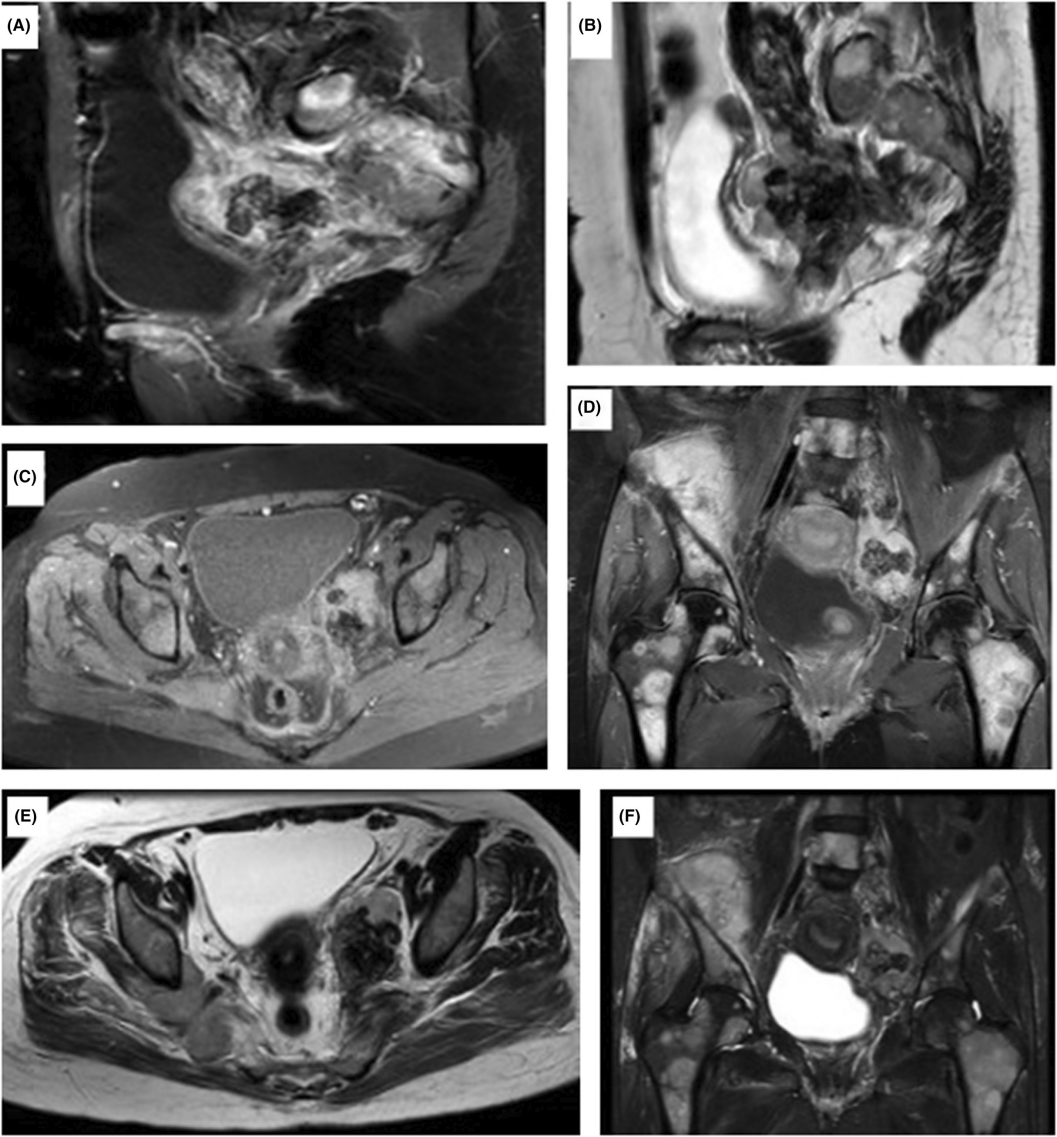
Coronal (A) and sagittal (C) contrast‐enhanced images revealed peripheral moderate enhancement of the non‐calcified components of the mentioned retroperitoneal mass and its extension to the left iliac vein complex as well as multiple enhancing bony metastases. The coronal STIR (B) and sagittal T2‐weighted (E) images can well display the extension of the mass to the expanded ipsilateral internal, external and common iliac veins. Axial T2‐weighted (F) and axial precontrast T1‐weighted (D) MR images represent a lobulated heterogeneous left paracervical mass with central popcorn signal voids (calcifications).
